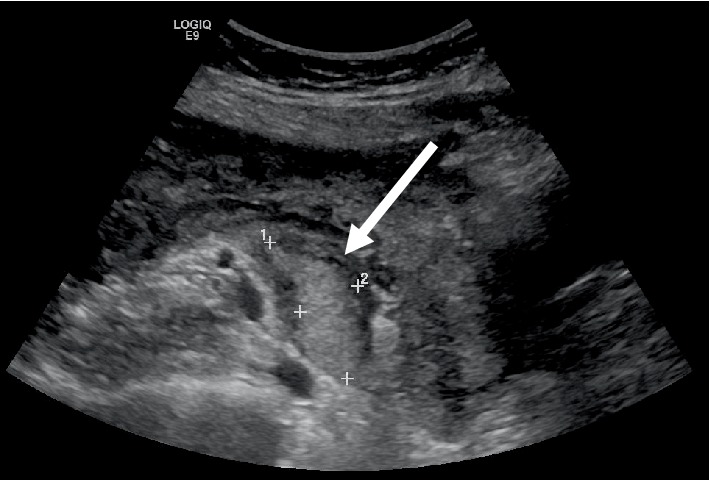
Ultrasonography of pelvis: the right ovary with an echogenic mass (arrow) measuring 4.2 × 2.1 × 3.8 cm is identified.
